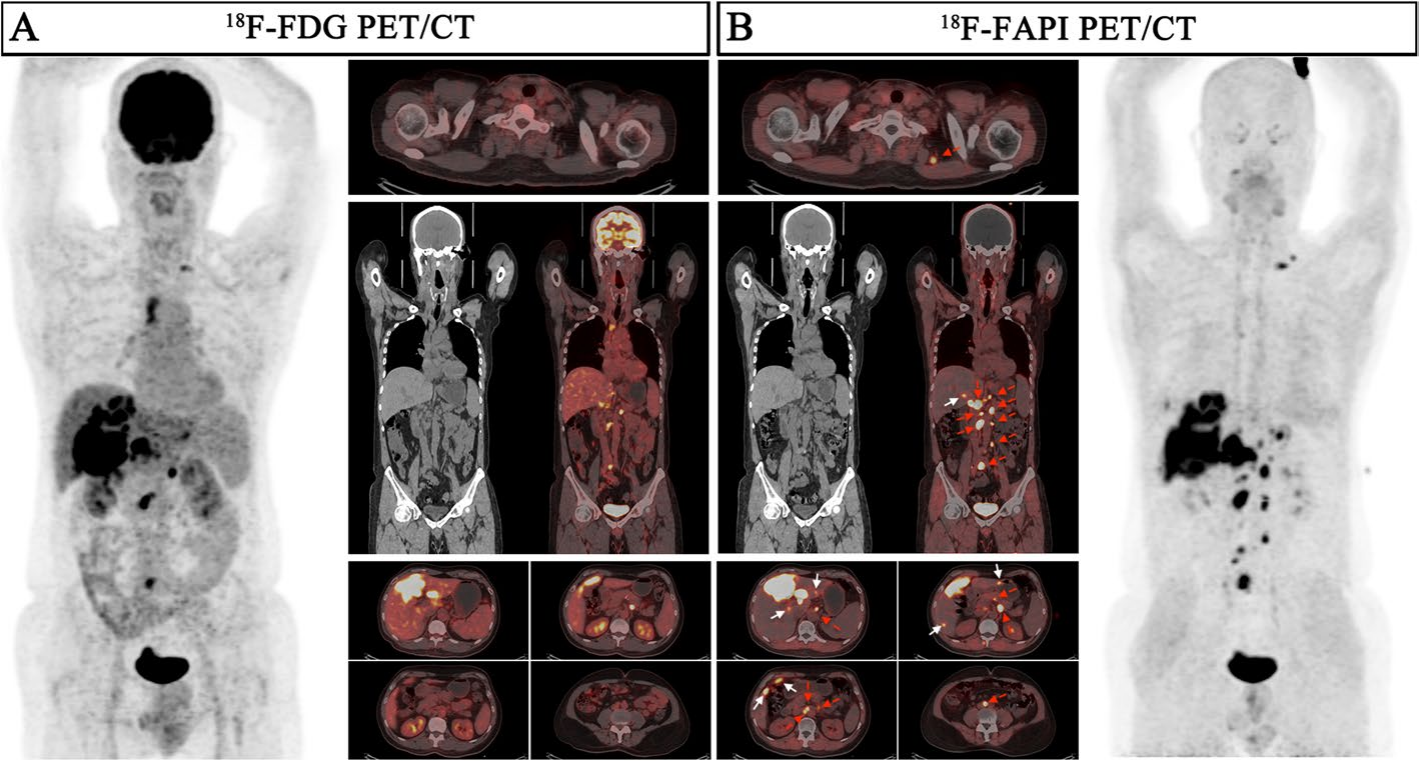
A 62-year-old male patient (Patient No. 13) with HCC (moderately differentiated) was confirmed by biopsy. Compared with ^18^F-FDG PET/CT, ^18^F-FAPI PET/CT revealed more intrahepatic subfoci (white arrow in axial images) and more lymph node metastases (red arrow in axial, coronal images). There was a lymph node in right upper mediastinum, showing low-uptake in ^18^F-FAPI but intense uptake in ^18^F-FDG, final pathological findings confirmed inflammatory
